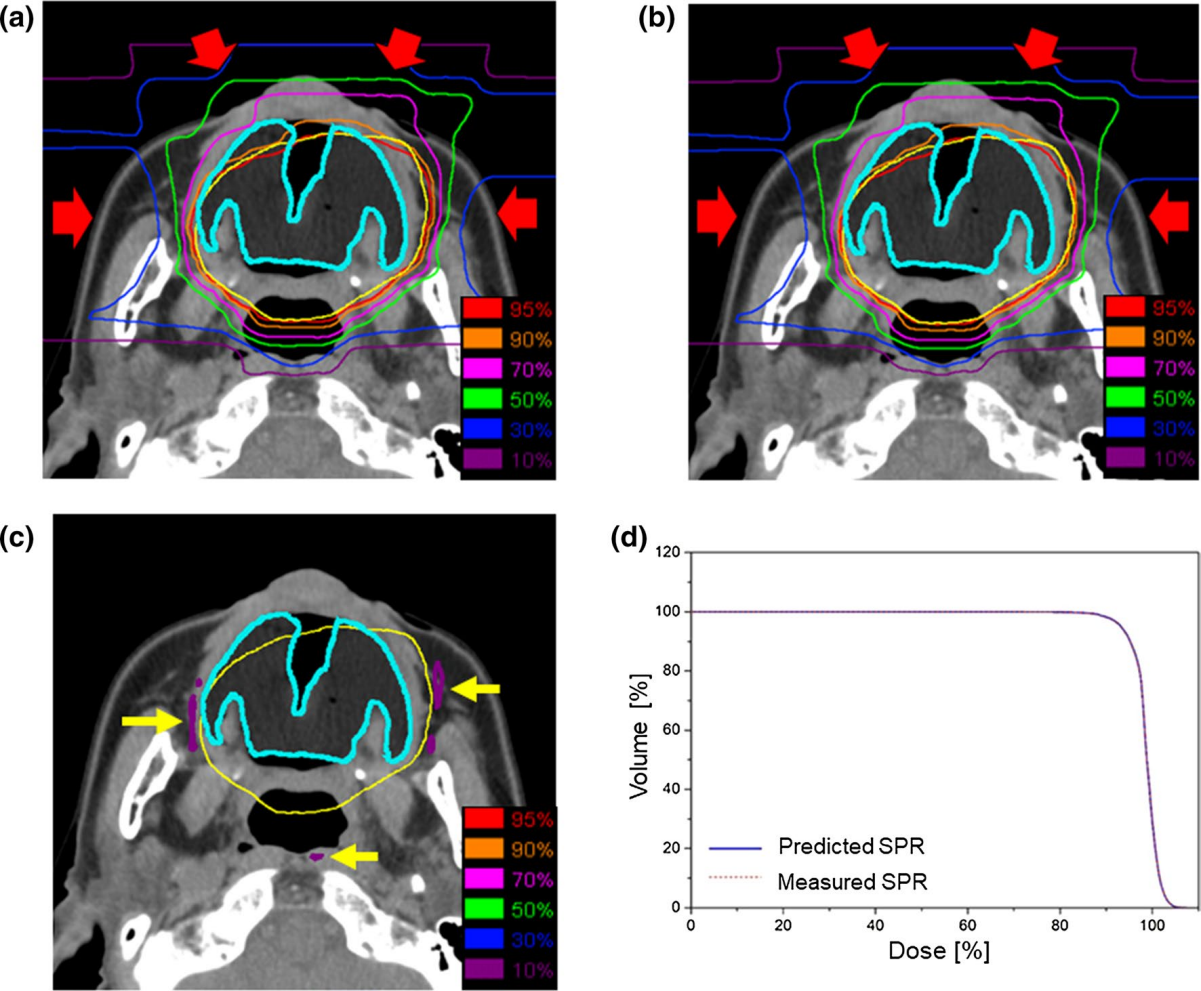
Axial computed tomography (CT) images with dose distribution and dose-volume histogram in head and neck cases irradiated by a passive irradiation method with a beam passed through the mouthpiece. Carbon-ion radiation therapy was delivered at 57.6 Gy (relative biological effectiveness) using four ports. The shown isodose lines correspond to 95%, 90%, 70%, 50%, 30%, and 10% dose areas. The planning target volume and mouthpiece are demarcated by yellow and cyan lines, respectively. a Dose distribution from predicted stopping-power ratio (SPR) calculated from the CT numbers of the mouthpiece using the CT number-to-SPR conversion table. b Dose distribution recalculated by assigning the SPR of the mouthpiece with the SPR of 1.00 obtained in the present study. c The differential dose distribution. There was a dose difference around the distal beam end between the planned and recalculated dose distributions (arrows). d Dose-volume histograms of the planning target volume. Dose-volume histogram calculated from the CT number of the mouthpiece with the SPR determined by the CT number-to-SPR conversion table (blue solid line). The dose-volume histogram was recalculated by assigning the SPR of the mouthpiece with the SPR 1.00 calculated in this study (red dotted line)
